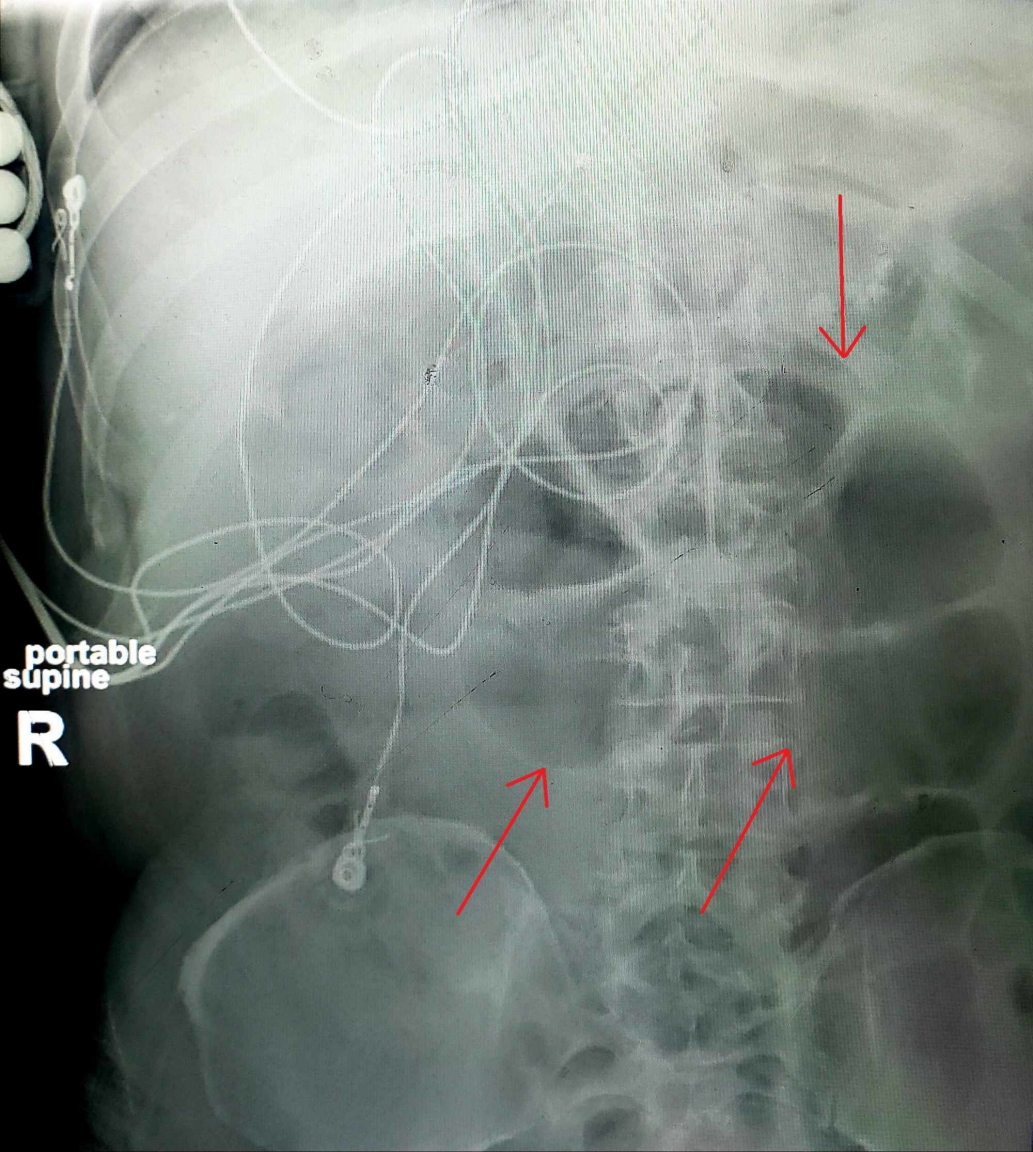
Abdominal X-ray. Red arrows show moderate stool burden with bowel distension.
